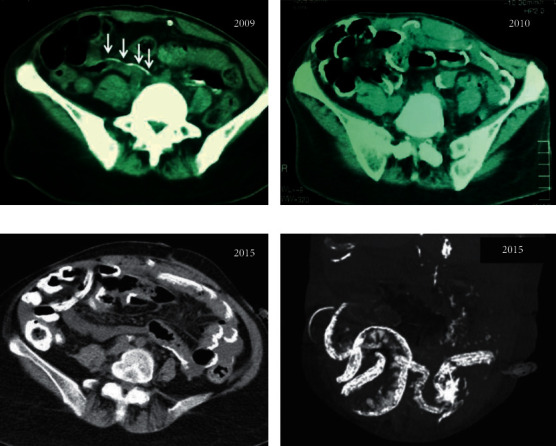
Abdominal CT scans of the patient in 2009 (a), showing mild linear peritoneal calcifications (arrows), which became full-blown calcifications of the visceral small bowel peritoneum a year later in 2010 (b) that remained or minimally increased over the next five years (2015 (c)). (d) Processed sagittal plane image of the 2015 abdominal scan showing the extent of bowel wall calcifications.
